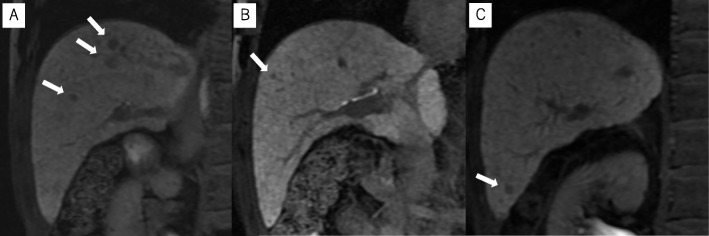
a–c Magnetic resonance imaging revealed low nodular shadows in the liver cell phase in the anterior segment and S6
